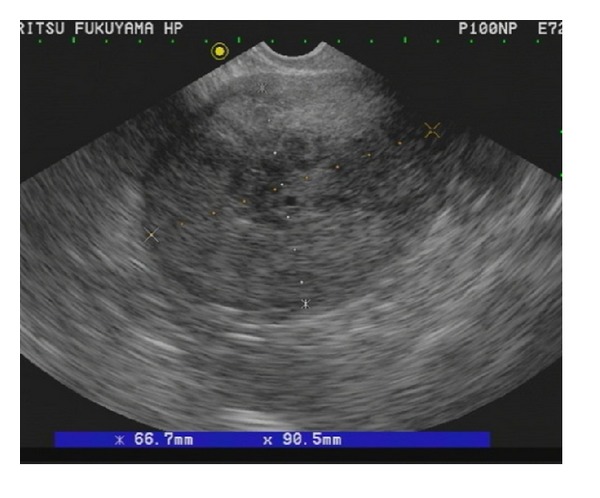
Ultrasound scans revealed a large heterogeneous mass occupying the whole uterine cavity.
